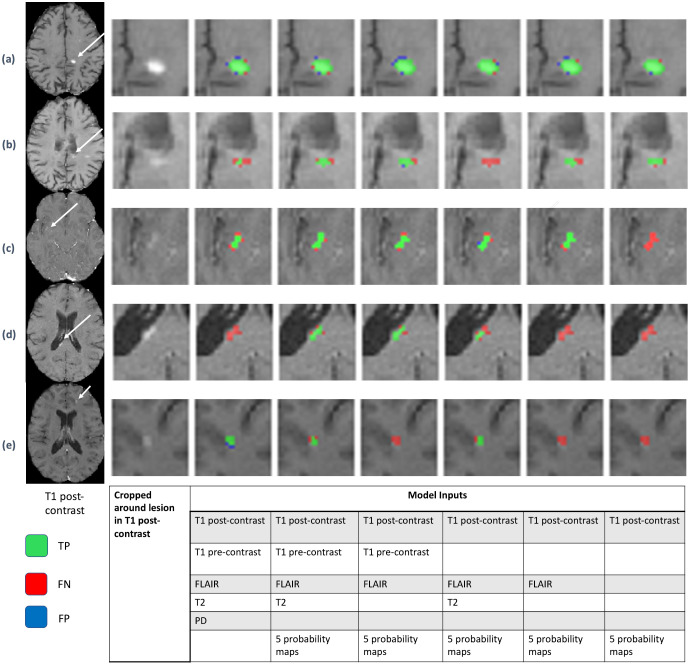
Predicted images for different input sequences. Each row represents image slices from different MRI sequences. The first column represents T1 post-contrast images and the second column represents the enlarged T1 post-contrast images around the lesion of interest. The other columns are performances of UNet and random forest model for different input sequences, which are tabulated below. The red regions are false negative segmentation, green is an overlap, and blue regions are false positives. The white arrow shows the lesion of interest. The first row (a) shows an example of lesion detection using any input while the second row (b) shows change in lesion segmentation volume due to different input. The third row (c) shows a lesion that cannot be detected using only T1 post-contrast. The fourth row (d) shows an example of lesion detection where T1 pre-contrast, T1 post-contrast and FLAIR are most influential to detect. The fifth row (e) shows a lesion that cannot be detected without T2.
